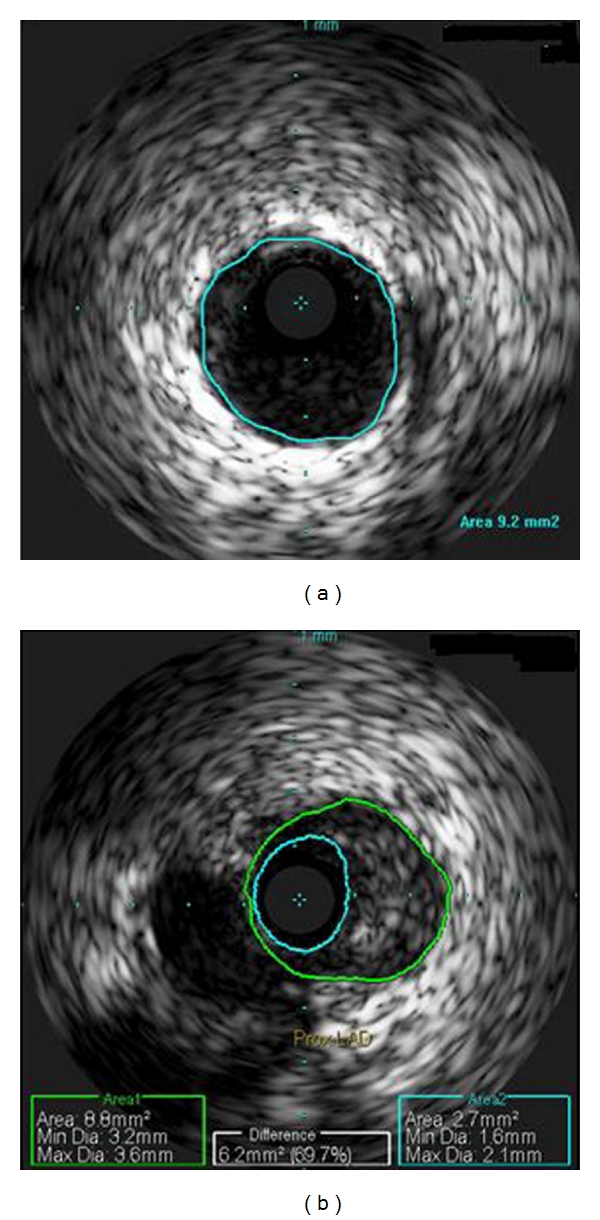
Intravascular ultrasound image of the left anterior descending artery; panel A represents the health proximal reference segment; panel B is the lesion which demonstrates negative remodeling.
